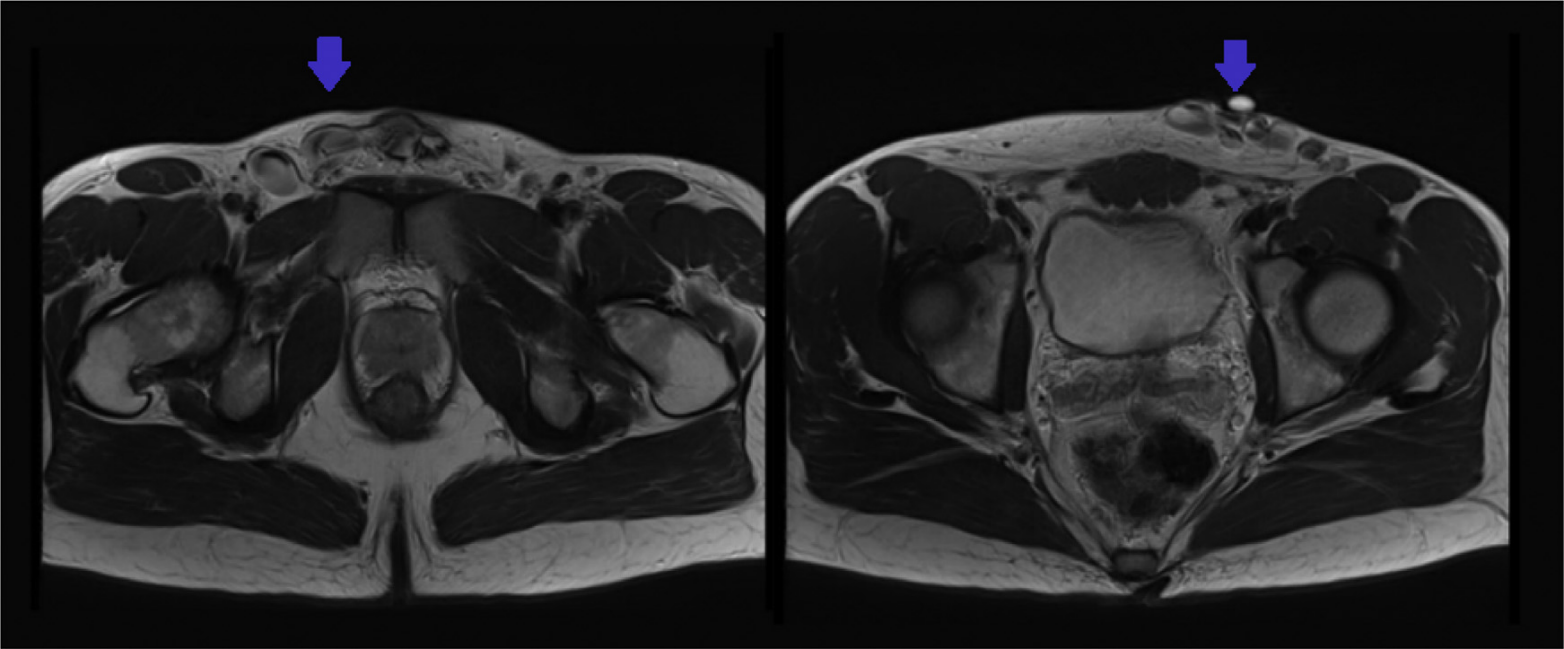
T2-weighted magnetic resonance venogram (MRV) of pelvis showing elaborate suprapubic collateral vessels (*arrow*) in both still frames in an axial projection.
